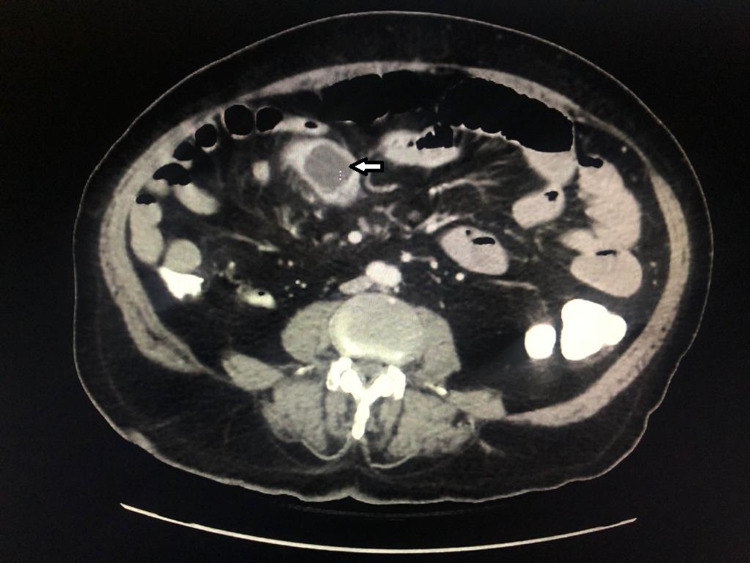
Abdomen CT with intravenous and oral contrast showing dilated bowel loops with a sigmoid mass
